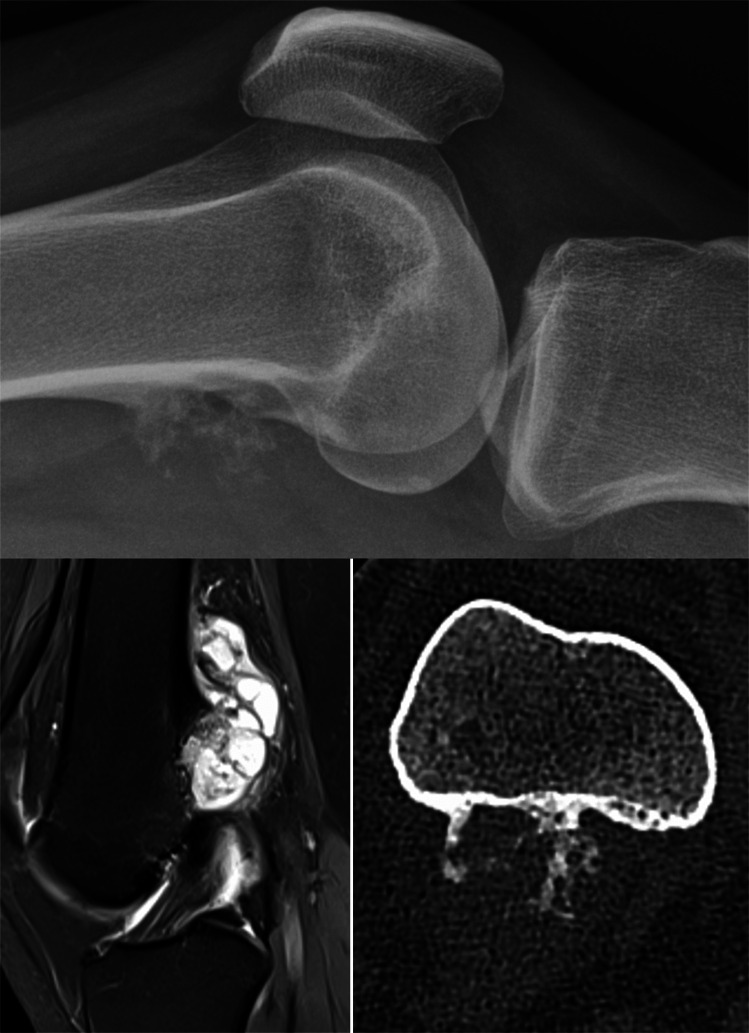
Twenty-four-year-old female with periosteal chondrosarcoma. Lateral radiograph (top) demonstrates a mineralised surface lesion with subtle cortical erosion and an associated cortical buttress. Sagittal PDFS image (bottom left) reveals a lobular subperiosteal surface lesion with chondral signal characteristics extending beyond the margins of the superior cortical buttress. Erosion of the underlying cortex is present without significant intramedullary extension. Axial CT image (bottom right) shows a low attenuation surface lesion with regions of intralesional matrix mineralisation and incomplete peripheral mineralisation. Adjacent sclerosis and deep cortical erosion that can be associated with chondrosarcoma are not seen
